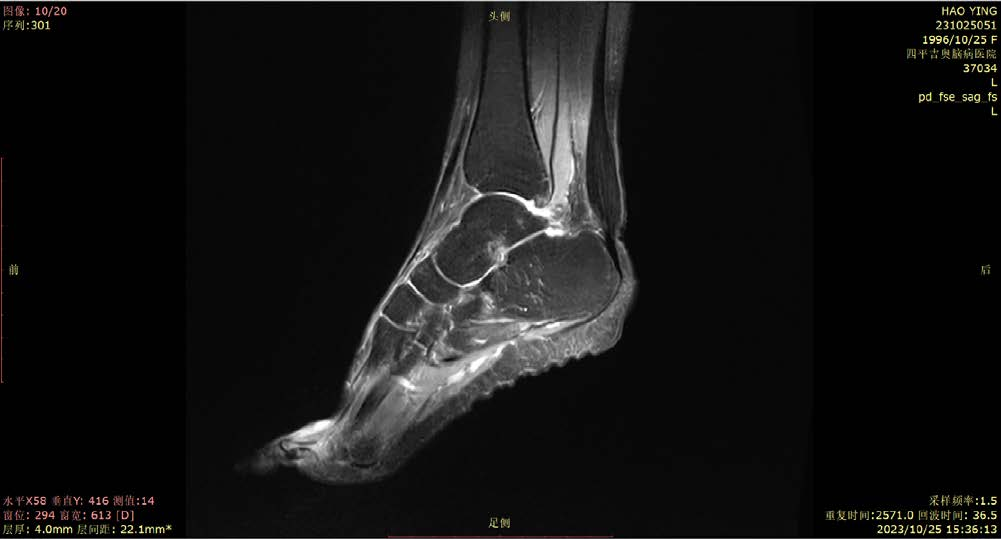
MRI proton weighted sequence of the patient’s Achilles tendon presenting an Achilles tendon mass. MRI, magnetic resonance imaging. MRI = magnetic resonance imaging.
